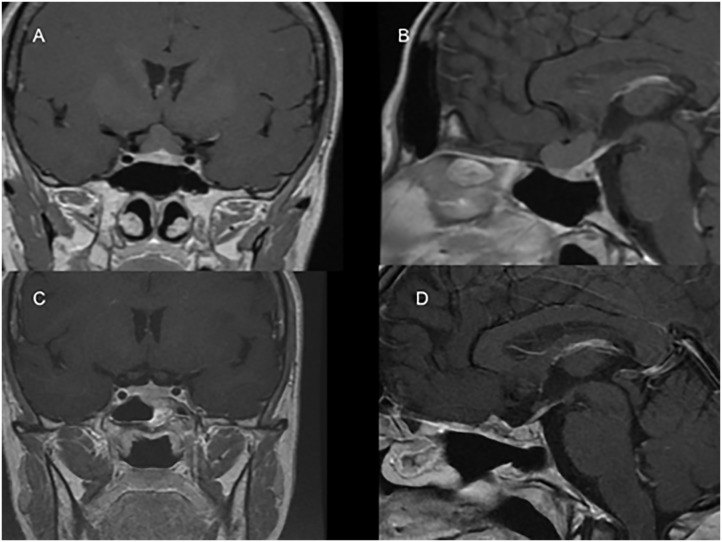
MRI scan showing a purely suprasellar Rathke’s Cleft Cyst before and after the surgical removal via an endoscopic endonasal approach (case showed in Fig 3). (A-B) Sagittal and coronal T1 weighted scans showing the lesion before being removed. The colloid has an isointense signal. (C-D) Sagittal and coronal post-gad scans after three months showing the cyst removal. It is possible to identify the optic chiasm the pituitary stalk and the normal pituitary gland.
